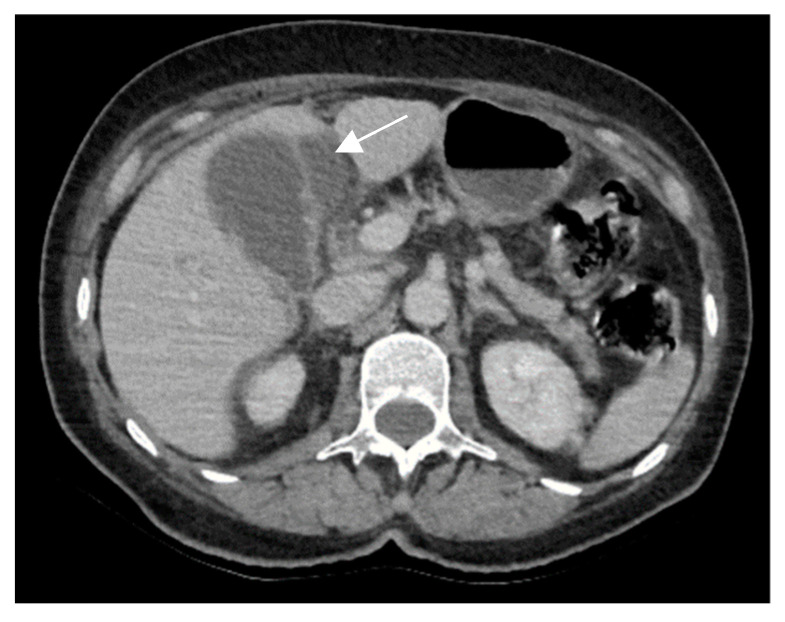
A 67-year-old woman undergoing contrast-enhanced CT for suspected abdominal acute appendicitis. CT images show pericholecystic abscess (white arrow) and symmetric wall thickening suggestive of acalculous cholecystitis.
